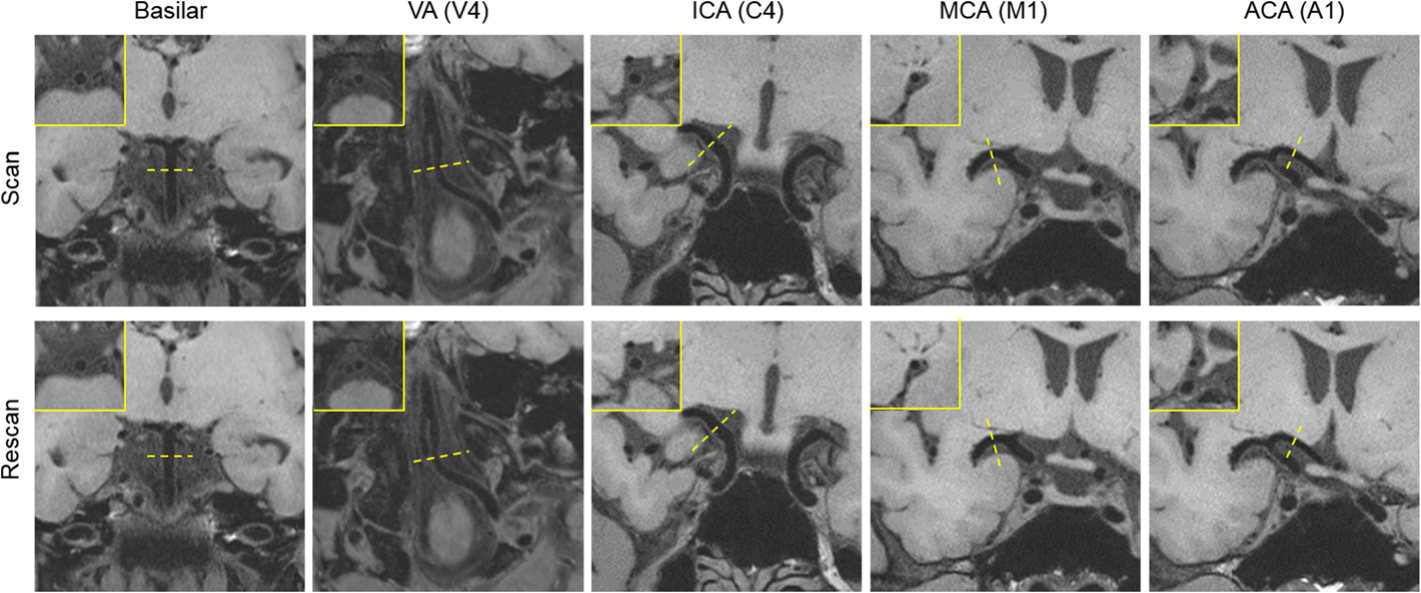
Representative 3D intracranial vessel wall MR images acquired in two scans from a 55-year-old male healthy subjects and reformatted cross-sections (upper left in the yellow box) for each designated arterial segment in the location indicated by the dashed lines. Both scans provide exquisite vessel wall depiction for the five designated arterial segments: the distal basilar artery, distal vertebral artery (VA (V4)), the distal internal carotid artery (ICA) supraclinoid segment (C4), the middle cerebral artery (MCA) M1 segment right after the trifurcation, and the anterior cerebral artery (ACA) A1 segments
